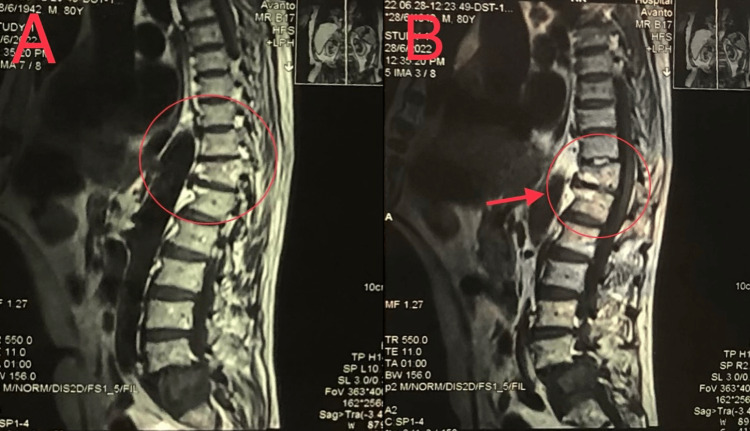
(A) and (B) images showing different MRI parasagittal sections of the spine with myelography representing abnormal signal intensity with anterior wedge compression in D11 and D12.
